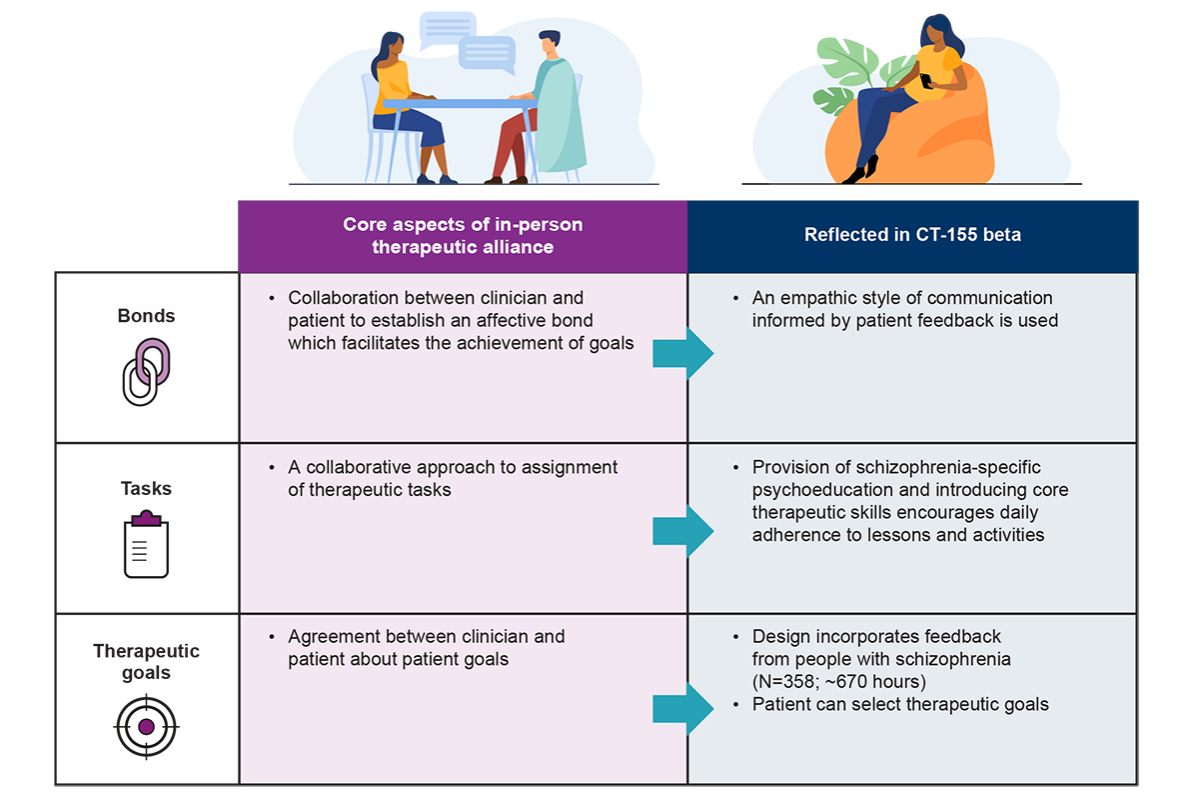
CT-155 beta captures aspects of in-person therapeutic alliance. Individual components of CT‑155 beta are designed based on the underlying principles of face-to-face treatment. These therapeutic techniques are intended to work synergistically to treat ENS and are supported by personalized engagement functionality that works in concert with the core therapeutic mechanisms. DWA: digital working alliance; ENS: experiential negative symptoms.
